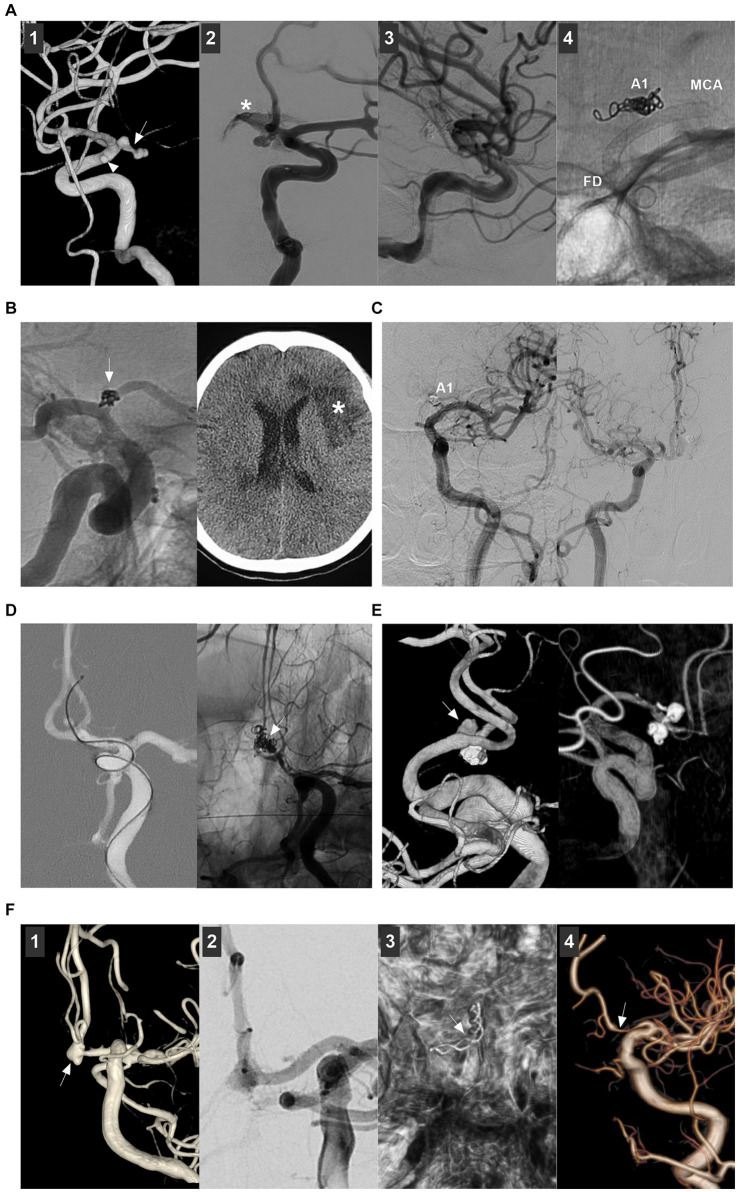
Complications of endovascular treatment for proximal ACA and AcomA aneurysms. (A) Panel 1: 3D-DSA showing an A1 aneurysm (arrow) and an anterior choroidal aneurysm (arrowhead); Panel 2: DSA showing the aneurysm rupture during the catheterization, the asterisk indicated contrast agent extravasation; Panel 3: DSA showing that the bleeding stopped by continuous coiling; Panel 4: X ray film showing the coiling in A1 segment origin, the flow diverter covered the A1 origin to avoid the aneurysm rerupture. (B) Left panel: DSA showing that the A1 aneurysm (arrow) was coiled; Right panel: Postoperative CT showing the ischemia (asterisk). (C) Left panel: Follow-up DSA showing that the asymptomatic occlusion of A1 segment after coiling the A1 aneurysm; Right panel: Follow-up DSA showing that A1 segment had sufficient blood flow from contralateral ACA. (D) Roadmap DSA showing that the AcomA aneurysm was perforated by the microcatheter (arrow); Right panel: Unsubtracted DSA showing the aneurysm was coiled (arrow). (E) Left panel: 3D-DSA showing a *de novo* AcomA aneurysm (arrow); the previous coiled aneurysm was below. Right panel: Six-month follow-up 3D-DSA showing that the aneurysm had no recurrence. (F) Panel 1: 3D-DSA showing a AcomA aneurysm (arrow); Panel 2: DSA showing the AcomA aneurysm was covered by flow diverter; Panel 3: Xper-CT showing the flow diverter (arrow); Panel 4: Six-month follow-up DSA showing that the aneurysm was cured and the proximal ACA was stenotic (arrow). 3D, three-dimensional; ACA, anterior cerebral artery; AcomA, anterior communicating aneurysm; CT, computed tomography; DSA, digital subtracted angiography.
